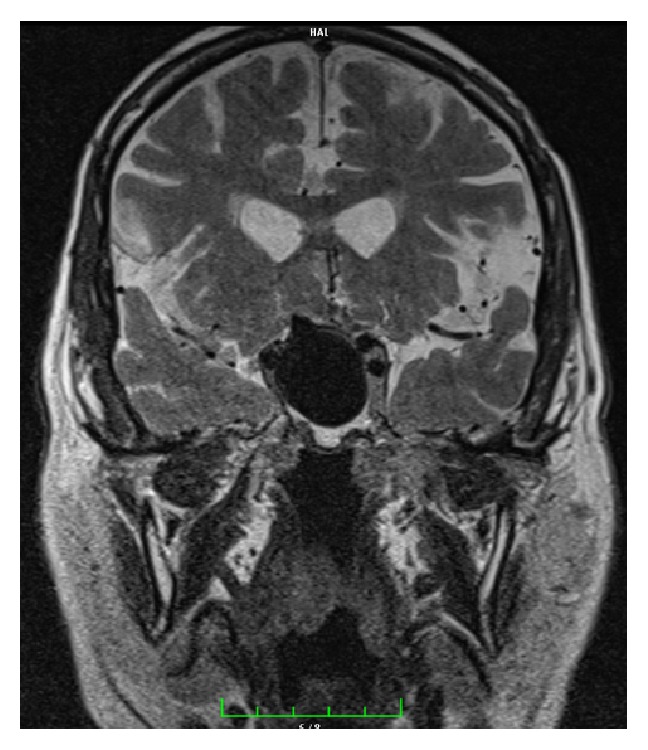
Coronal T2-weighted MR image showing giant saccular aneurysm.
